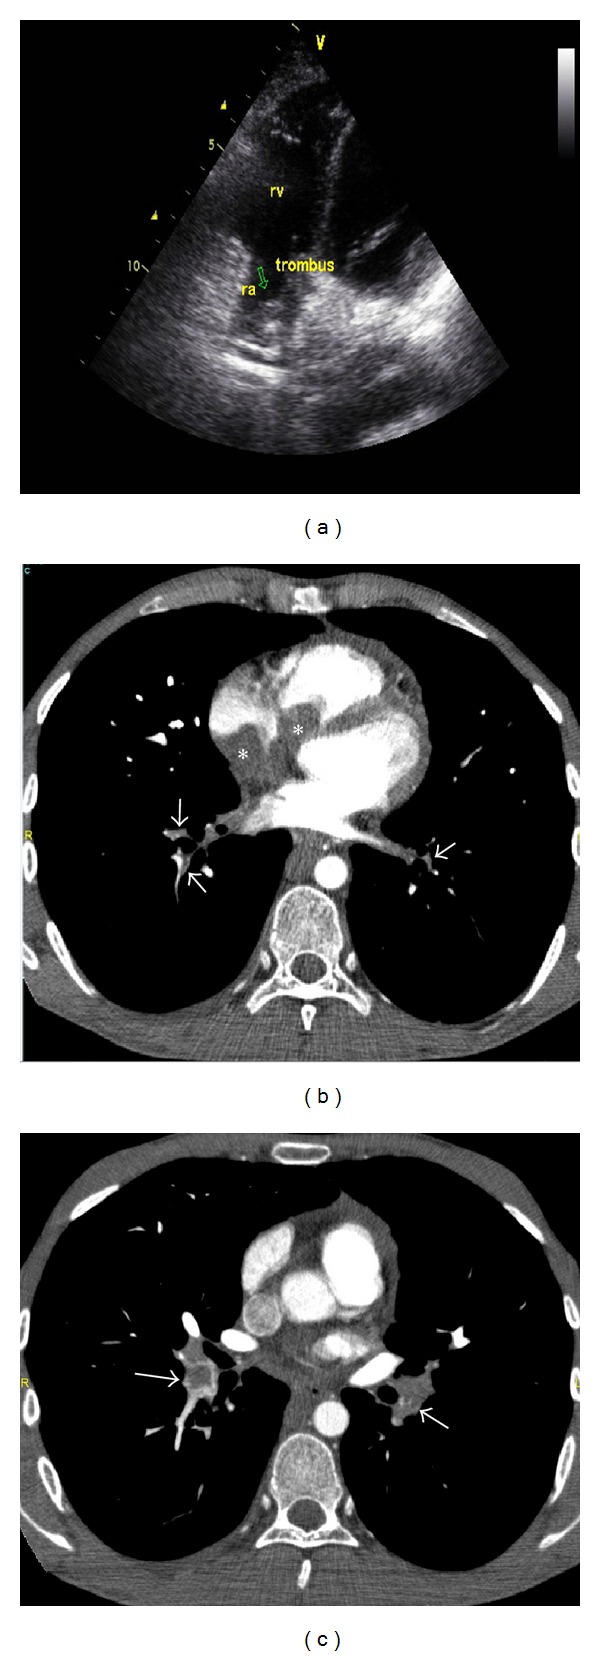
(a) Transthoracic echocardiography showed a mobile mass filling the half of the right atrium (4.5 × 3 cm in size) (arrow) extending the right ventricule (1.5 × 1.6 cm in size) and into the vena cava. (b) Contrast-enhanced CT angiographic image shows a large filling defect in the right atrium extending into the right ventricle (asterisks). Segmental arteries in both lower lobes are of small calibre due to chronic thromboembolism (arrows). Pulmonary hypertension resulted in straightening of the interventricular septum. (c) Both lower lobe arteries contain central filling defects (arrows) representing superimposed acute pulmonary embolism.
